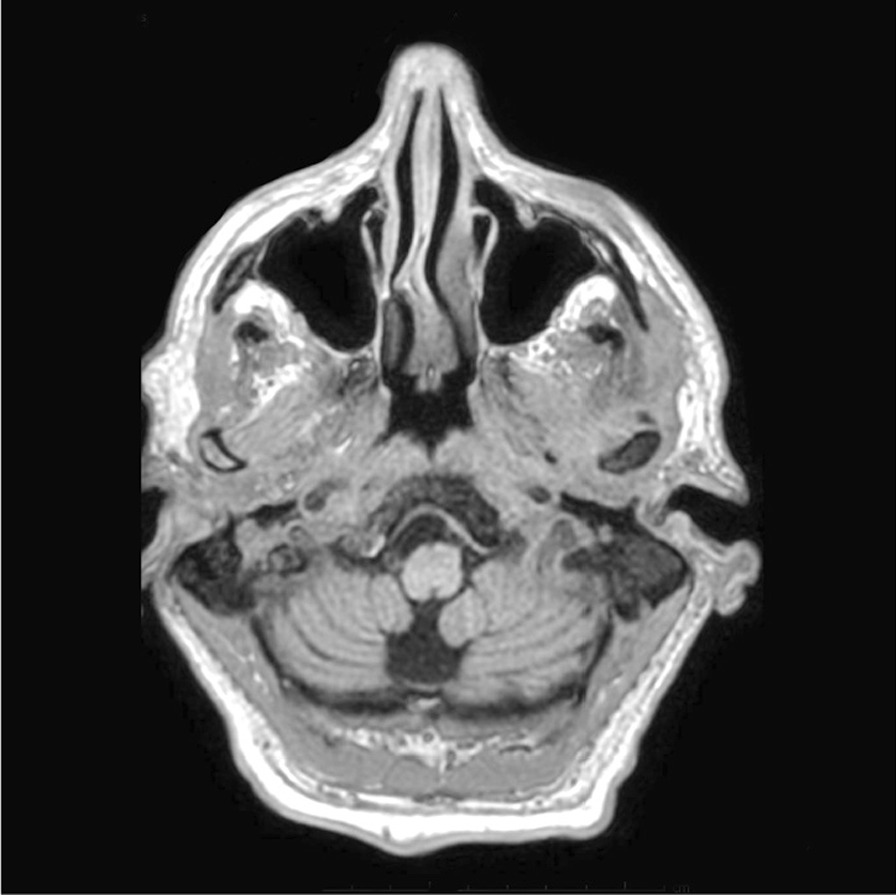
Following therapy with intravenous benzylpenicillin, a follow-up brain MRI 3 months later displayed a pronounced reduction in the size of the gumma (right)
